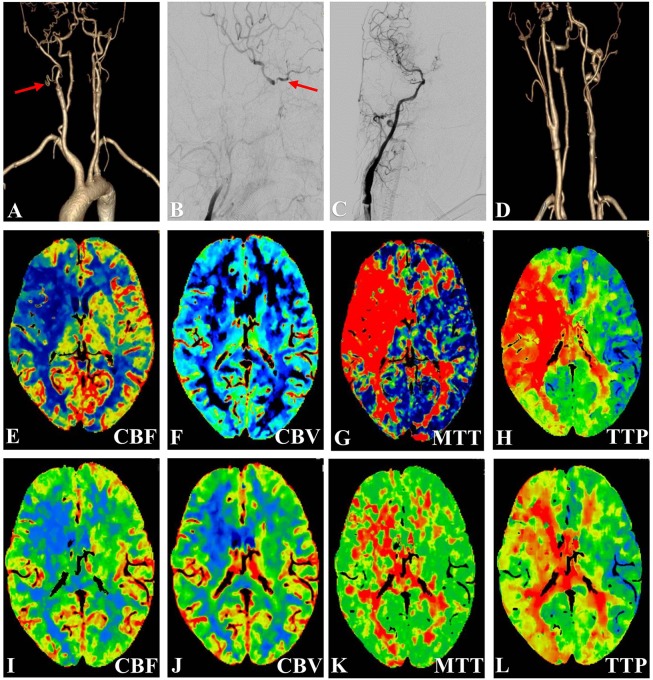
Representative Case 1. A 67-year-old woman with right-sided limb weakness underwent hybrid surgery for internal carotid artery occlusion (ICAO). (A) Preoperative computed tomography angiography (CTA) confirmed total occlusion (arrow) of the right ICA without a stump and (B) with filling at the ophthalmic segment. (C) Digital subtraction angiography (anteroposterior view) showed successful recanalization after deployment of the stent. (D) CTA showed a patent ICA at 3 months after the surgery. (E–L) Preoperative and postoperative perfusion CT. Postoperative perfusion CT showed improvements in the (I) cerebral blood flow (CBF), (K) mean transit time (MTT), and (L) time to peak (TTP) after recanalization [compared with low CBF in (E), high MTT in (G), and delayed TTP in (H)).
